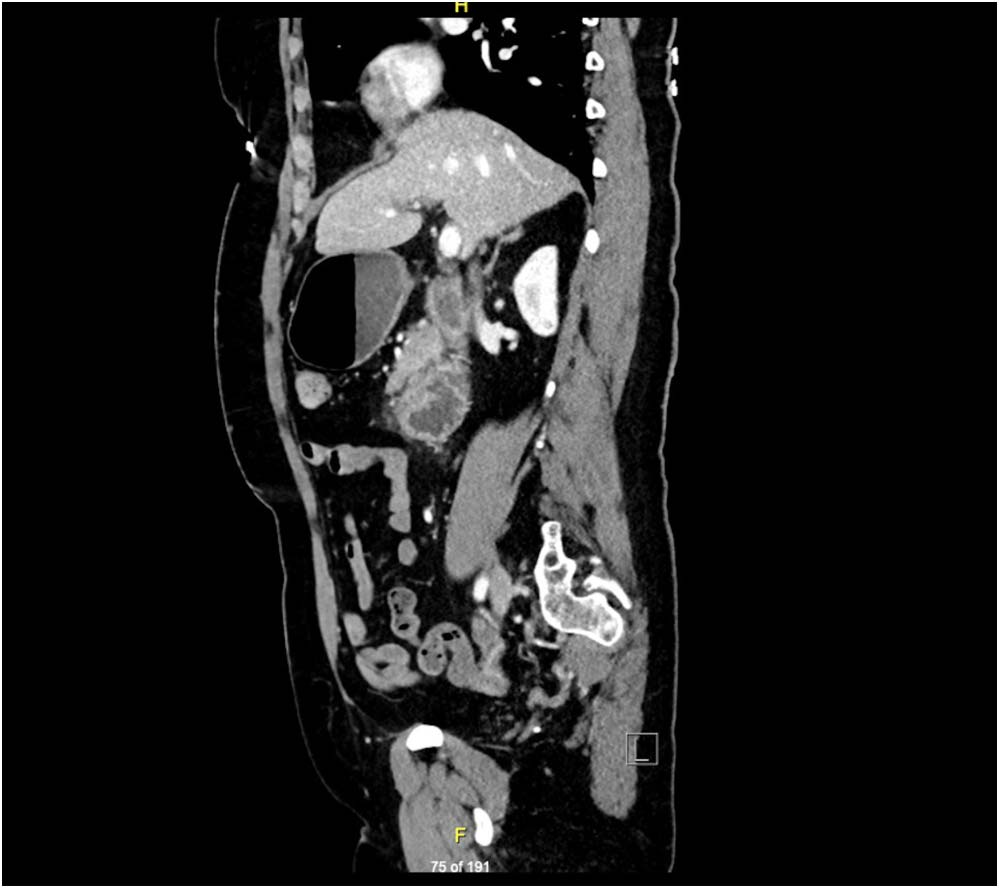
Sagittal view of a contrast-enhanced computed tomography scan of the abdomen showing stomach distension and a thickened, narrow segment of the third portion of the duodenum with surrounding enlarged lymph nodes.
